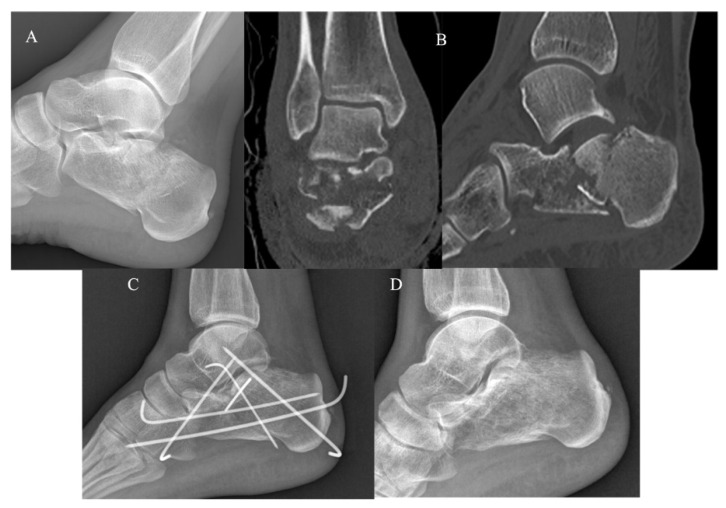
Mini-open approach and K-wire fixation. (A) Pre-operative radiographs; (B) pre-operative CT scan in coronal and sagittal plane showing a Sanders III CF; (C) post-operative radiograph control; (D) post-operative radiographs at 4-year follow-up.
